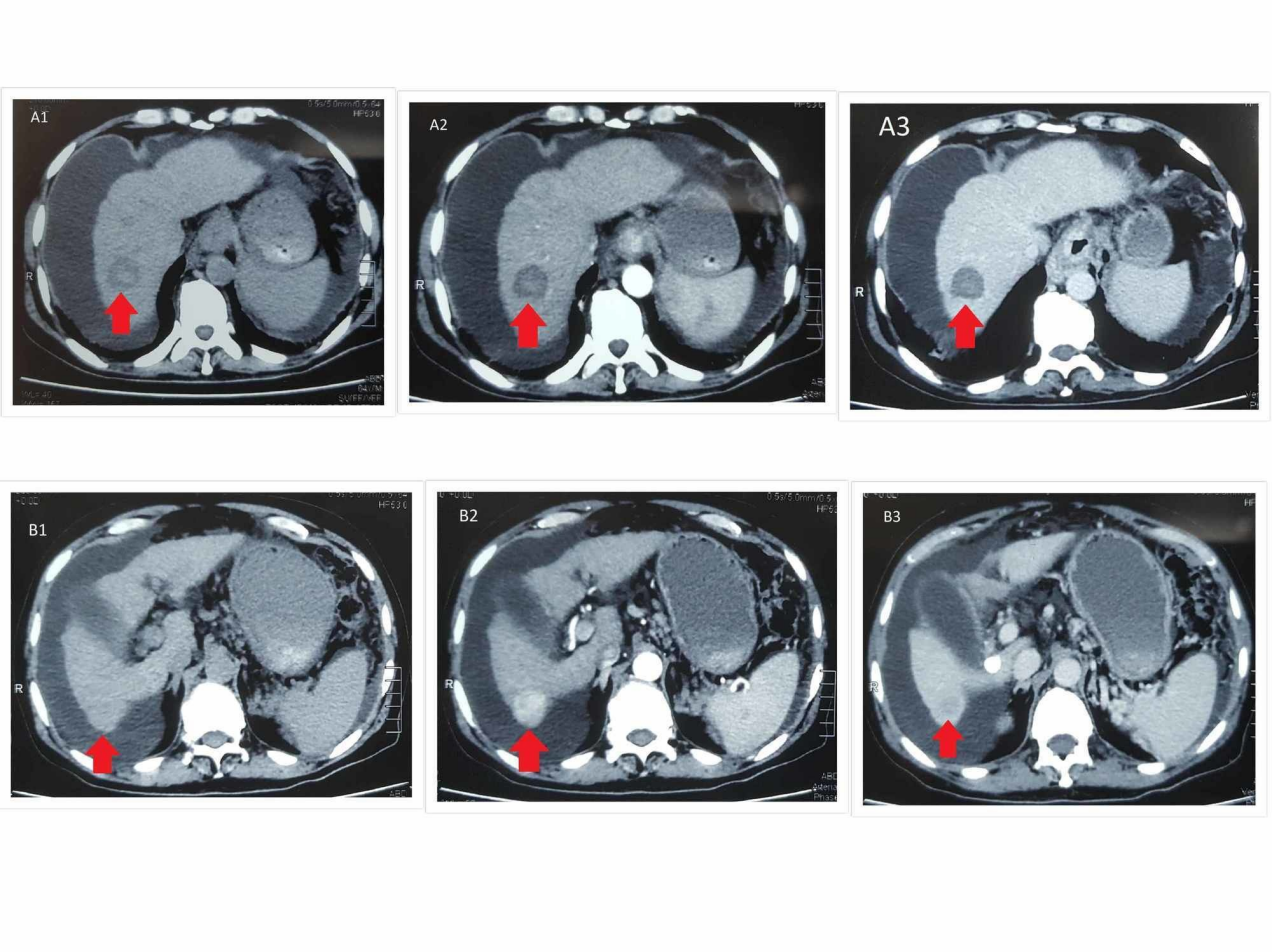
Triphasic CT abdomen Showing one lesion in segment VIII (A) and another in segment VI (B) of the liver in successive plain (A1/B1), arterial (A2/B2), and venous (A3/B3) phases of the study. A hypodense lesion (A1) in segment VIII was consistent with previous successful microwave ablation. This lesion is neither showing arterial hyperenhancement (A2) nor any washout (A3). A new arterialized lesion (B2) is seen in segment VI of the liver, showing washout of contrast on the venous phase (B3). No evidence of thrombosis in the portal vein or extrahepatic metastasis seen. Gross ascites is also evident. CT: computed tomography
